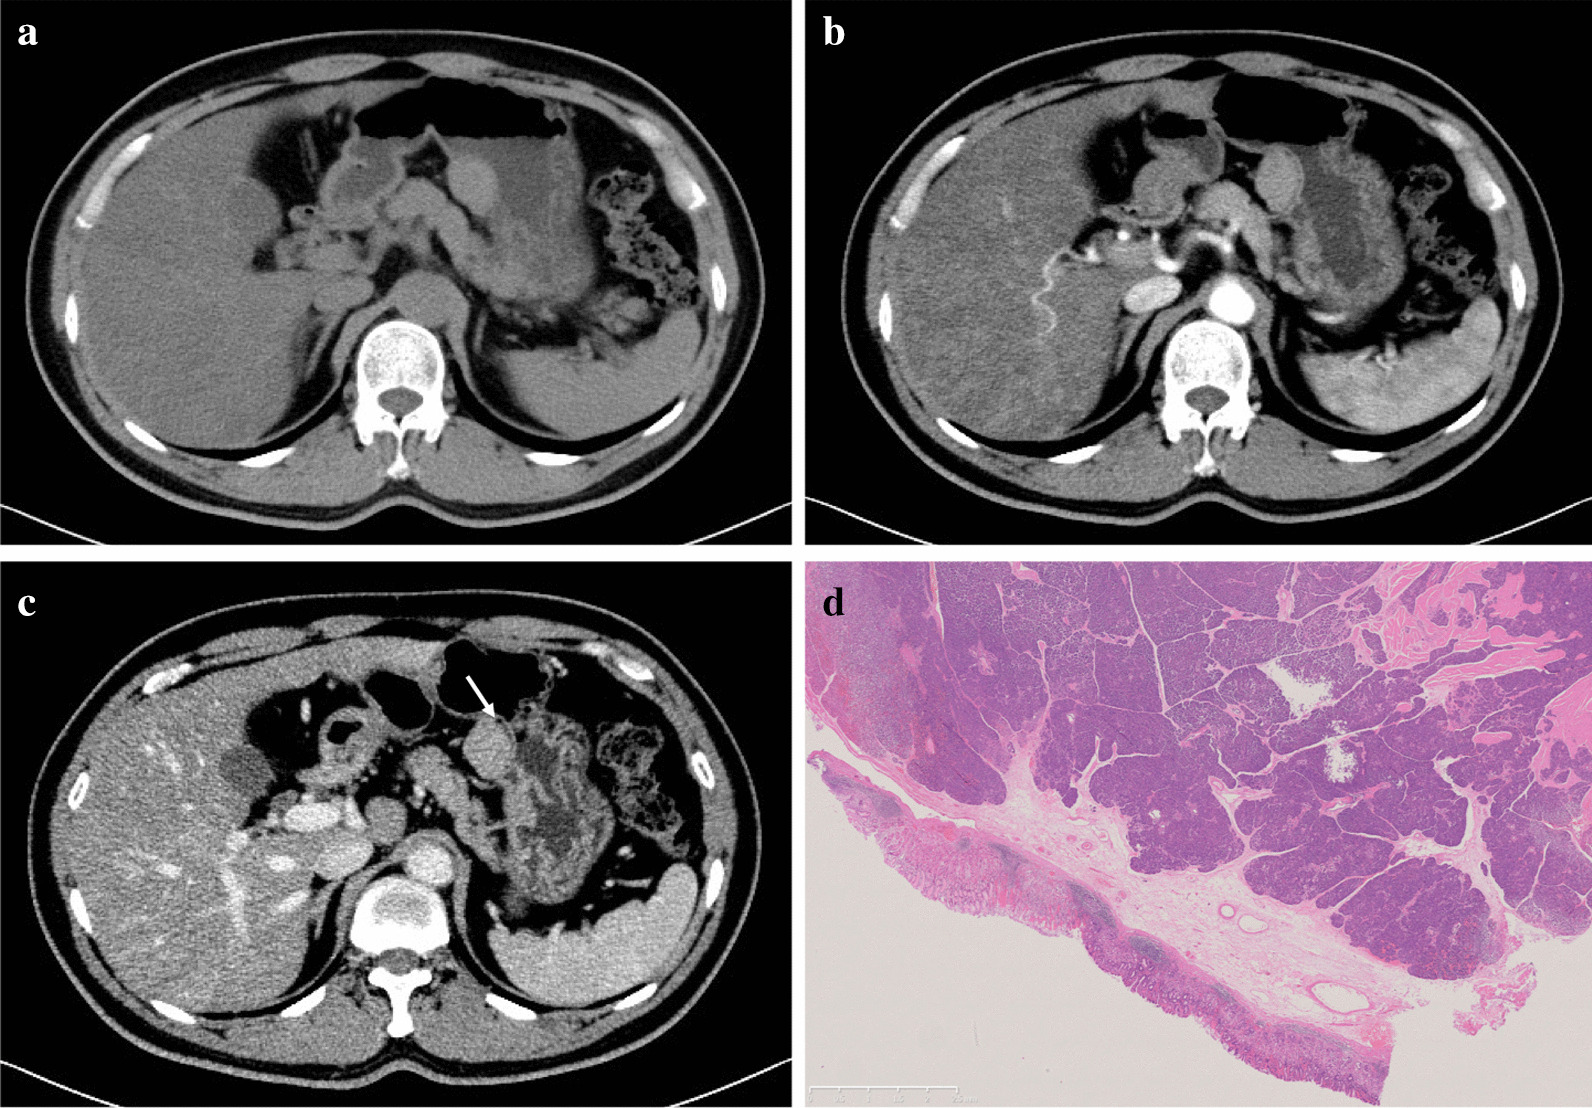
Patient 2, 40–50 years old, sex1. Ectopic pancreas in gastric body (a–c). Axial CT scans (non-enhanced, arterial and portal phase) show an oval Exophytic mass in the gastric body and also the fat-line of peritumor (arrow). The lesion shows homogeneous enhancement and equal attenuation to the pancreas (d). The lesion was confirmed as ectopic pancreas pathologically
